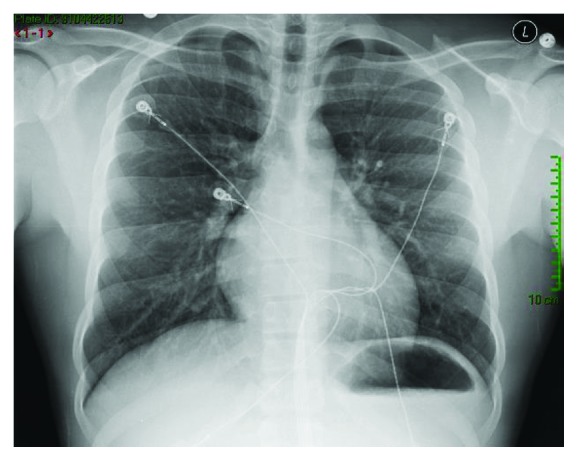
Chest X-ray did not reveal any significant radiographic abnormalities.
